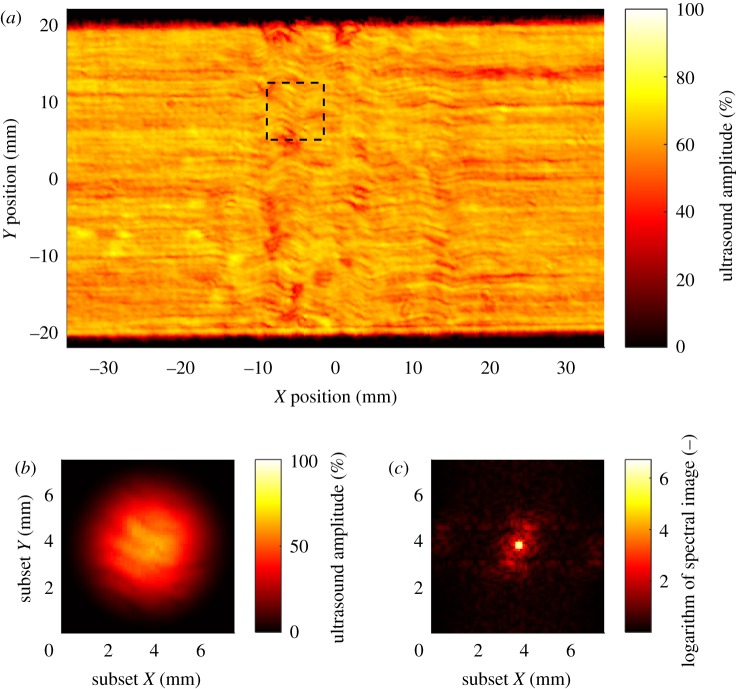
An amplitude C-scan of a 17.5% nominal waviness coupon showing the waviness in the top ply (*a*) with a subset of ultrasound data indicated by a dashed rectangle. A Hann window was applied to the subset (*b*) and its spectral image (*c*) used to determine the fibre orientation at the subset location.
